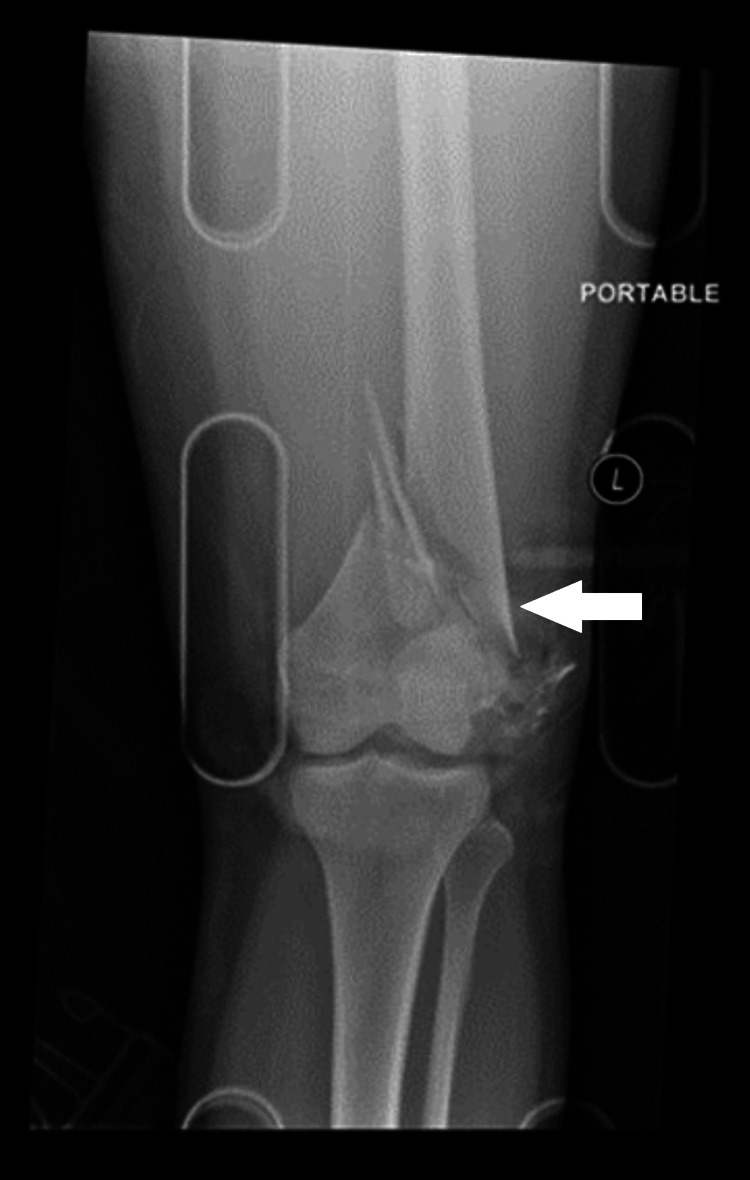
Initial injury AP X-ray image of the left knee revealing a comminuted fracture of the distal femur involving the distal diaphysis, which is shortened and extends laterally through the femoral condyle, as highlighted by the arrow. AP: Anteroposterior.
